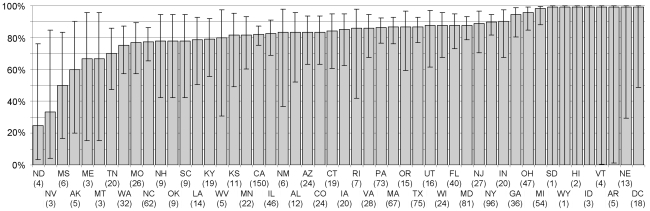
Percentage of positive results by US state. Percentage and 95% logit-derived confidence interval of papers published between 2000 and 2007 that supported a tested hypothesis, classified by the corresponding author's US state (sample size for each state is in parentheses). States are indicated by their official USPS abbreviations: AL-Alabama, AK-Alaska, AZ-Arizona, AR-Arkansas, CA-California, CO-Colorado, CT-Connecticut, DC-District of Columbia, FL-Florida, GA-Georgia, HI-Hawaii, ID-Idaho, IL-Illinois, IN-Indiana, IA-Iowa, KS-Kansas, KY-Kentucky, LA-Louisiana, ME-Maine, MD-Maryland, MA-Massachusetts, MI-Michigan, MN-Minnesota, MS-Mississippi, MO-Missouri, MT-Montana, NE-Nebraska, NV-Nevada, NH-New Hampshire, NJ-New Jersey, NM-New Mexico, NY-New York, NC-North Carolina, ND-North Dakota, OH-Ohio, OK-Oklahoma, OR-Oregon, PA-Pennsylvania, RI-Rhode Island, SC-South Carolina, SD-South Dakota, TN-Tennessee, TX-Texas, UT-Utah, VT-Vermont, VA-Virginia, WA-Washington, WV-West Virginia, WI-Wisconsin, WY-Wyoming. All US states were represented in the sample except Delaware.
